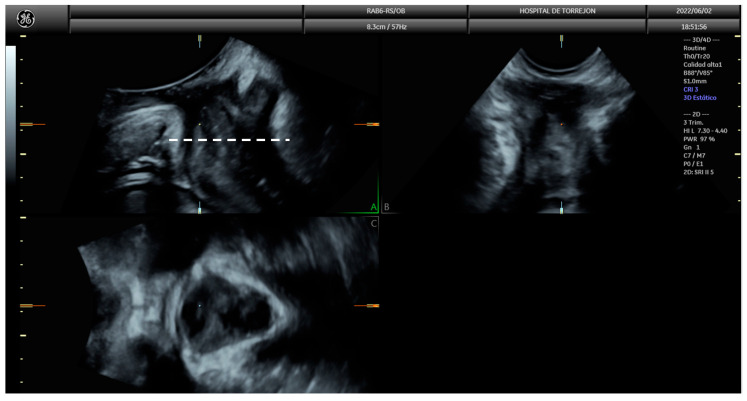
Multiplanar view of translabial pelvic floor ultrasound. Plane (A) displays the mid-sagittal plane acquired directly during the scan. The dashed white line indicates the location of the axial plane used for measuring the hiatal diameters and area. Plane (B) shows coronal plane and plane (C) shows the 3D reconstruction of the axial view of the urogenital hiatus, where hiatal dimensions are assessed.
